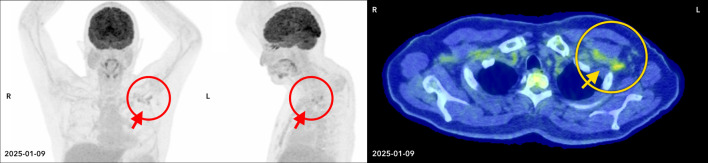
Post-BV-AVD imaging. Post-BV-AVD FDG positron emission tomography-computed tomography (PET-CT) maximum intensity projection (axial and coronal, left) and transverse section (right) showing interval reduction in nodal size and FDG uptake (indicated by arrows). BV-AVD: brentuximab vedotin, adriamycin, vinblastine, and dacarbazine; FDG: fluorodeoxyglucose.
